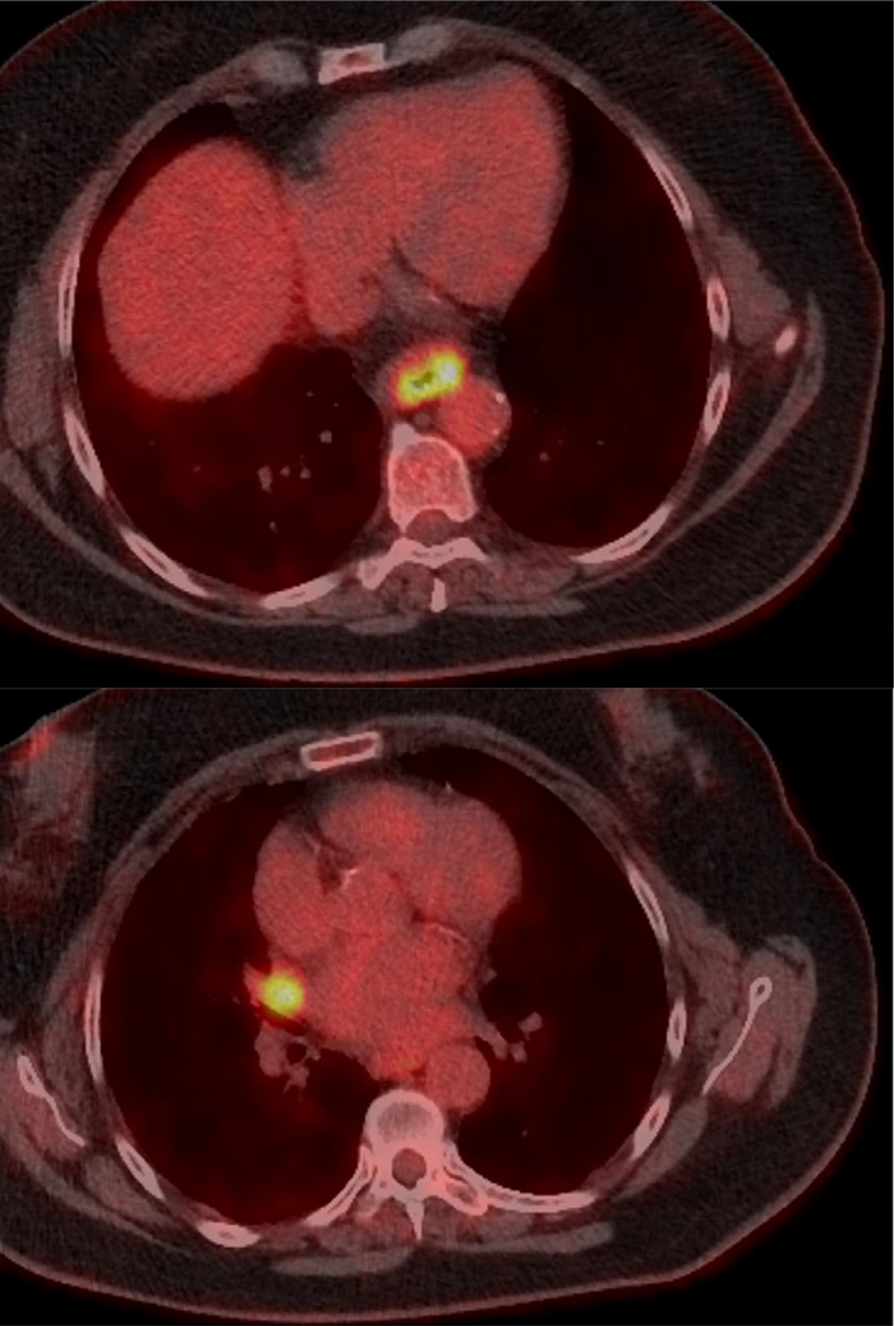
PET CT scan demonstrating uptake within the oesophageal mass (top) and the hilar mass (bottom).
